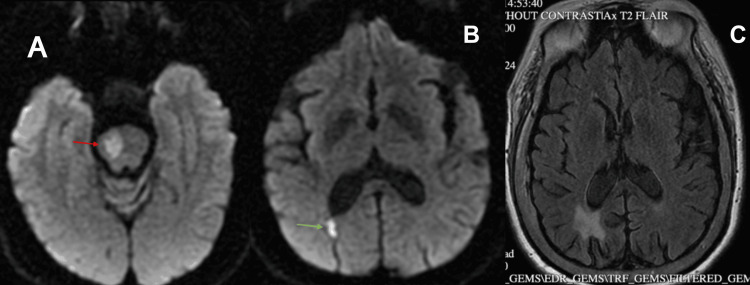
MRI showing areas of acute stroke A: Brain MRI, DWI demonstrating acute infarct in the right paramedian pons (red arrow). B: Brain MRI, DWI demonstrating posterior parietal periventricular infarct (green arrow). C: FLAIR imaging demonstrating periventricular white matter changes. DWI, diffusion-weighted imaging; FLAIR, fluid-attenuated inversion recovery
